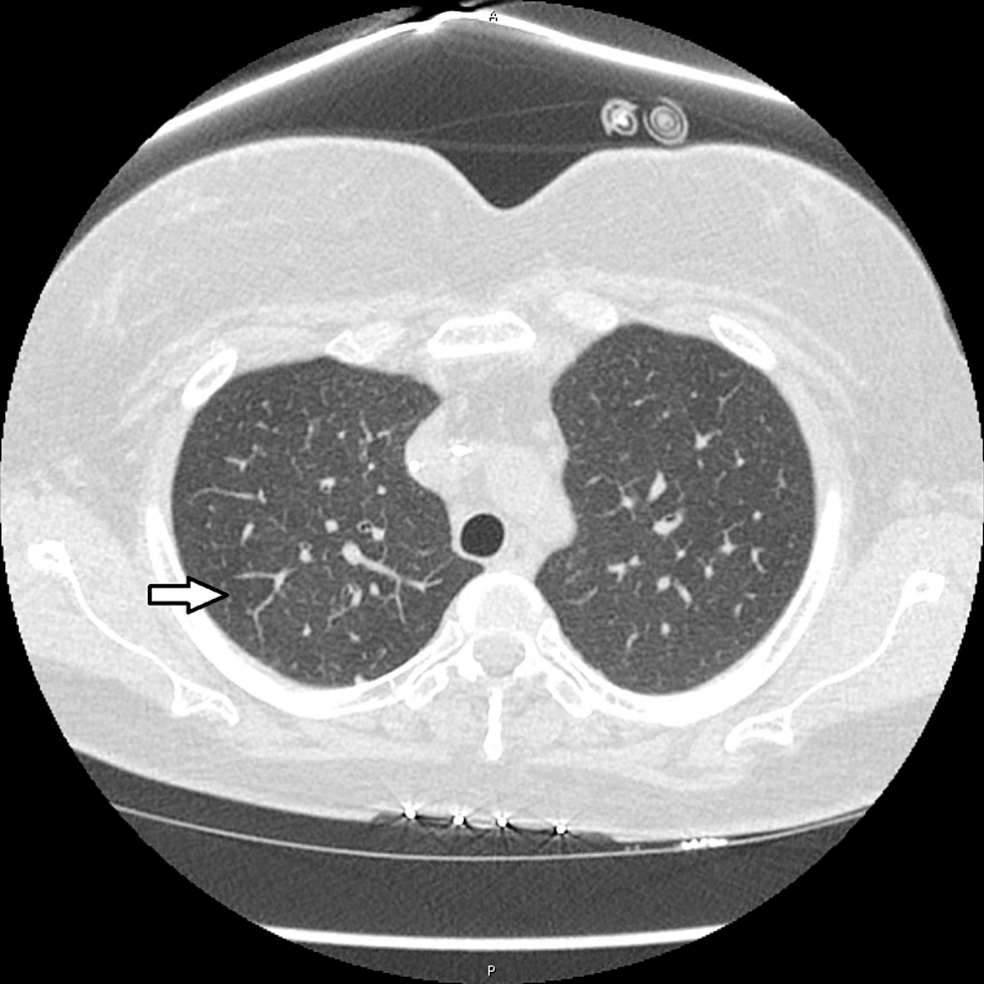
CT of the chest. Lung window. Lung upper lobes. The improvement of the right upper lobe tree in bud opacities (arrow). Compare with Figure 1.
